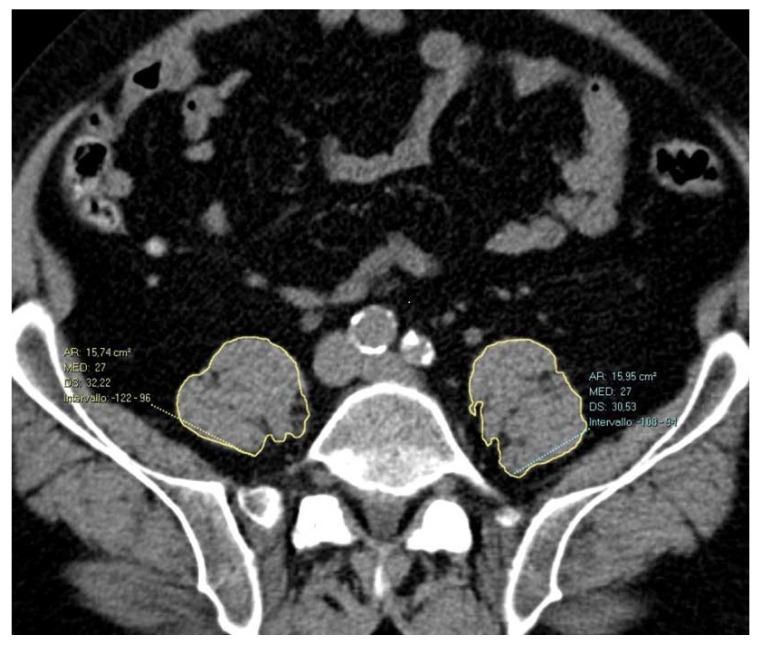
Axial non-enhanced CT scan showing polygonal manual segmentation (yellow outline of bilateral psoas muscles). The right psoas muscle area is 1574 mm^2^, and the left psoas muscle area is 1595 cm^2^. The PMD is 27 HU on both sides.
